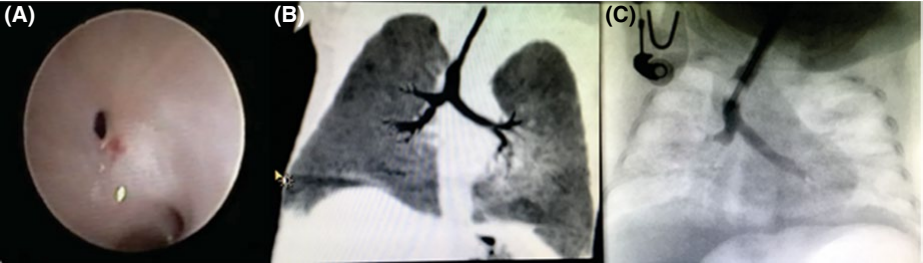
A, Bronchoscope image of the right main bronchus, demonstrating the pinhole size of the bronchus intermedius. B, CT‐scan image of the circumferential narrowing of bronchus intermedius just below the opening of the right upper lobe. C, Contrast study under fluoroscopy confirming the narrowing
